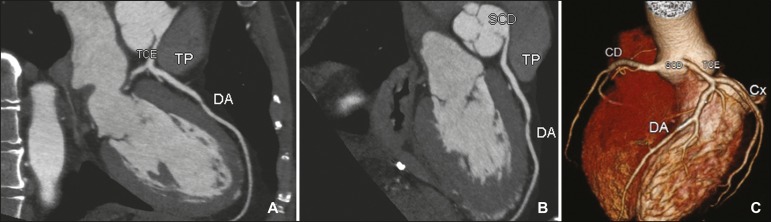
Contrast-enhanced CT. Coronal plane (A), oblique
